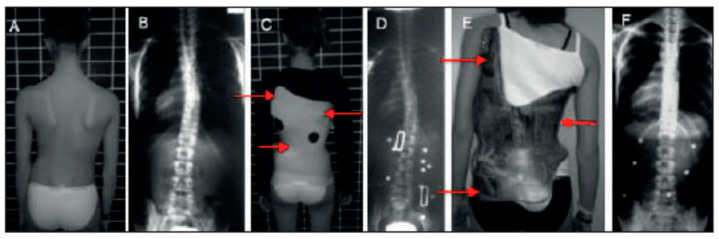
Three-point pressure brace principle. (A) clinical assessment of the scoliosis; (B) radiographic evaluation; (C) brace derotation point of action; (D) radiological assessment of the brace; (E) definitive brace; (F) radiological assessment during the follow-up.
